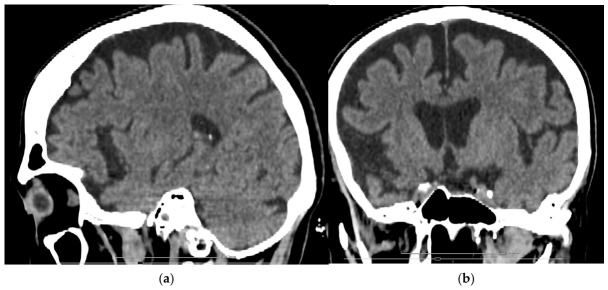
Brain CT findings: (a) sagittal view demonstrating predominant frontal and anterior temporal cortical atrophy; (b) coronal view showing bilateral frontotemporal volume loss with associated enlargement of the frontal horns/temporal horns.
